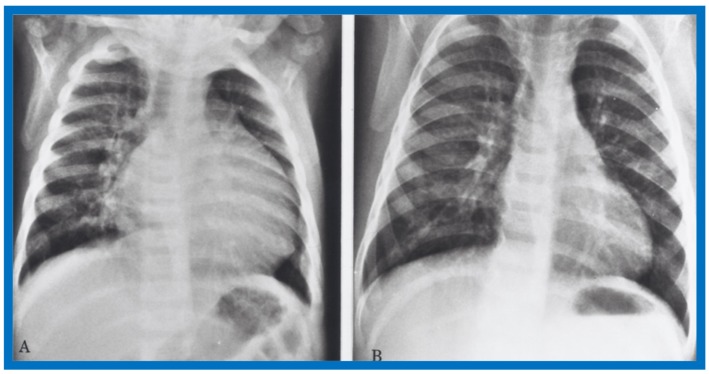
Antero-posterior view of chest x-rays prior to (A) and following (B) hydralazine therapy from a different infant. Moderate cardiomegaly with pulmonary venous congestion was seen prior to therapy (A) which improved remarkably after therapy (B), similar to that seen in Figure 42.
